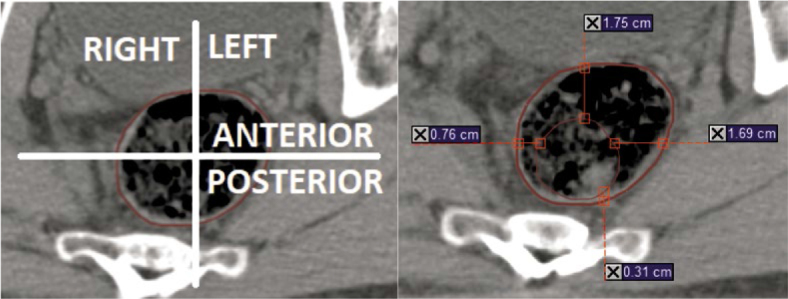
Direction of measurements taken and example of measurements used in the study. The bold brown line is the reference position on CT image; the thin brown line is the daily position on cone-beam computed tomography (CBCT)
